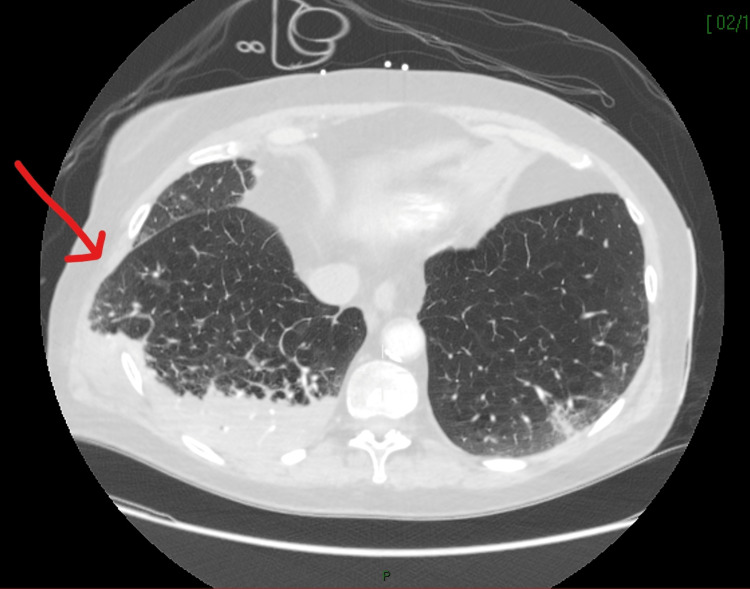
CT chest (axial view) demonstrating right lateral lung herniation and right lower lobe consolidation. Red arrow pointing to lung herniation.
